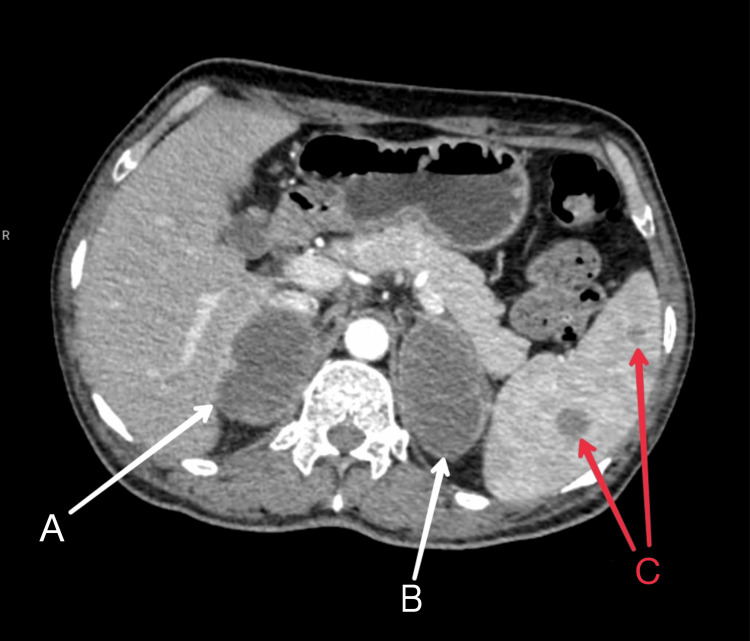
Axial contrast-enhanced CT images of the abdomen demonstrating (A) a heterogeneous right suprarenal mass (~6.3 × 4.3 × 9.3 cm; white arrow), (B) a heterogeneous left suprarenal mass (~6.8 × 4.2 × 9.8 cm; white arrow), and (C) multiple hypodense lesions involving all poles of the splenic parenchyma (red arrows)
